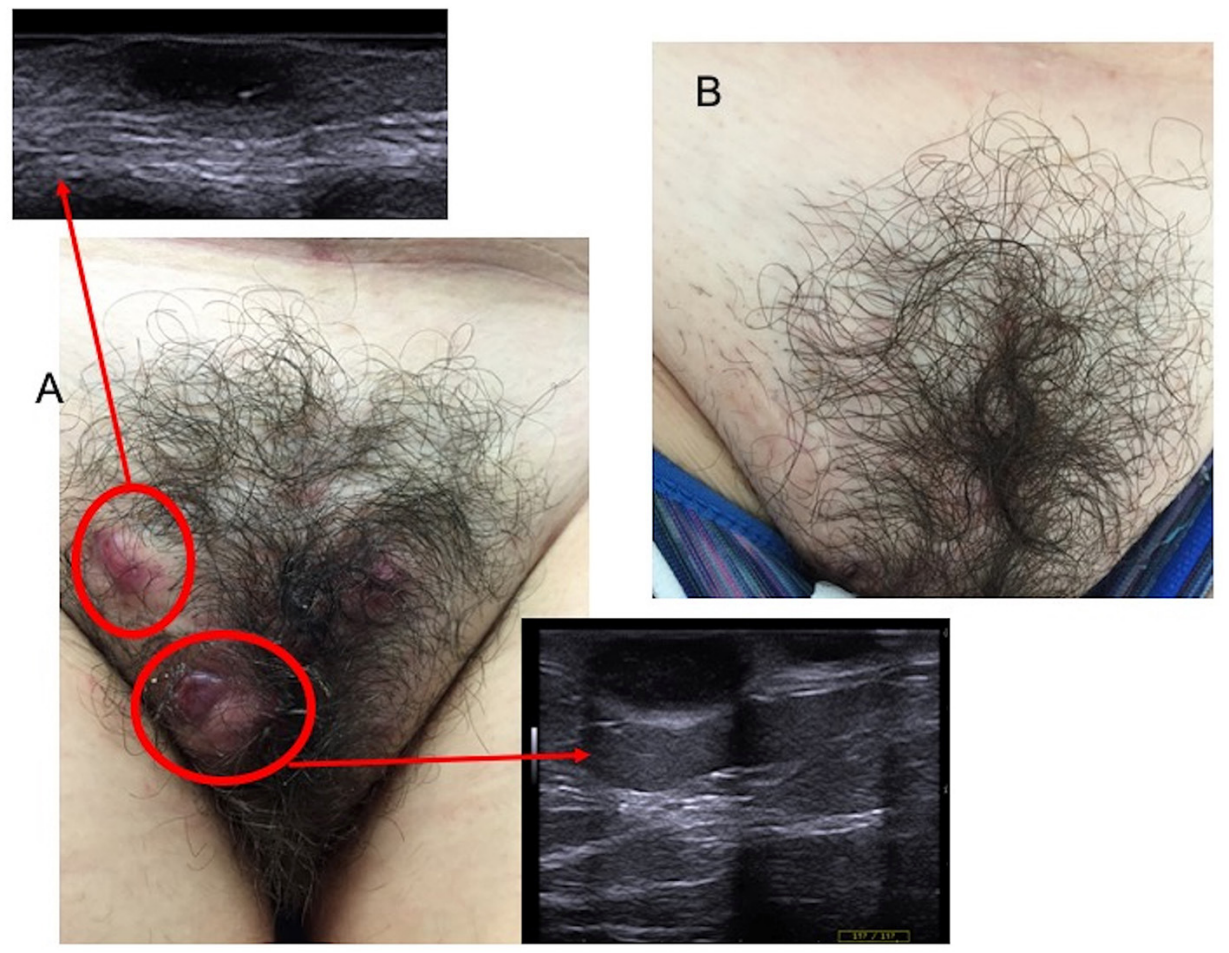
Nodular lesions of the groin with the ultrasound assessment before cryotherapy (A), and complete resolution at 3-month follow-up (B).
